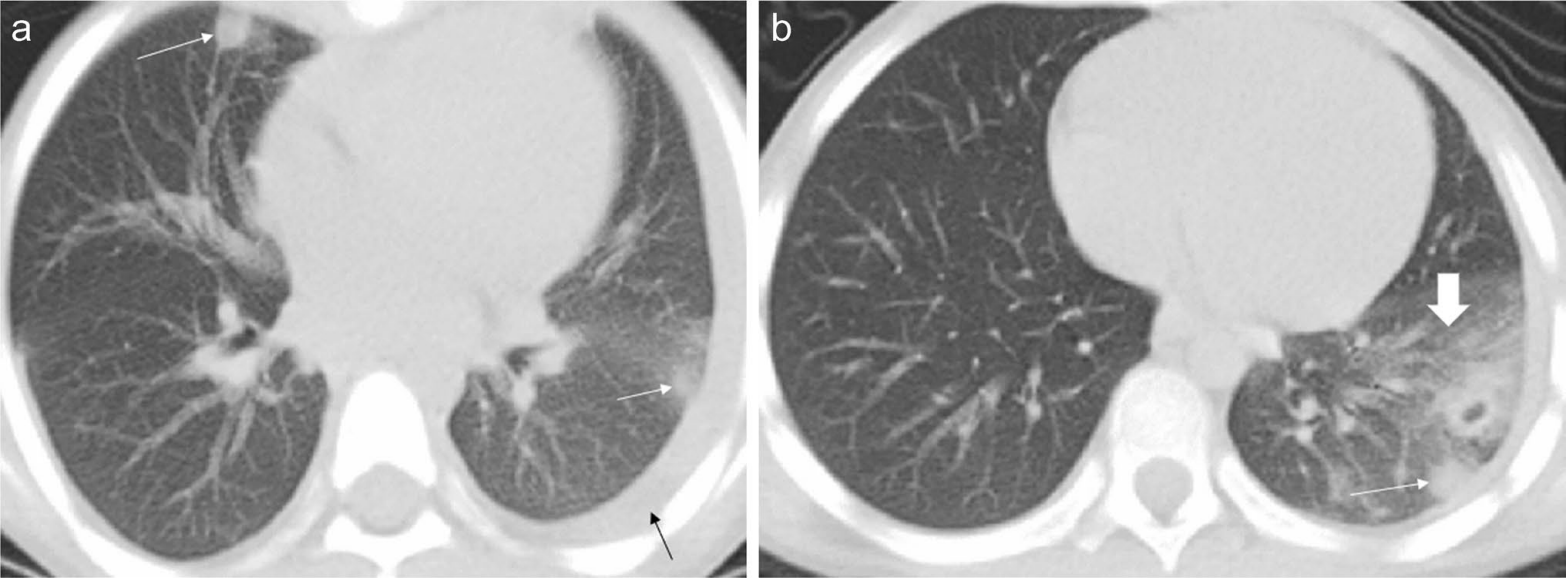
Axial non-contrast-enhanced lung window computed tomography images in a 7-year-old girl with paragonimiasis who presented to hospital with abdominal pain and subcutaneous nodules. a Image at the level of the mid lung zone shows ill-defined lung nodules (*white arrows*) and a left-sided pleural effusion (*black arrow*). b Image at the level of the lower lung zone shows ill-defined lung nodules (*thin arrow*) and alveolar opacification (*thick arrow*) (figure from “A retrospective clinical analysis of pediatric paragonimiasis in a Chinese children’s hospital from 2011 to 2019” by Qian et al.; licensed under CC BY 4.0; original cropped and annotated)
